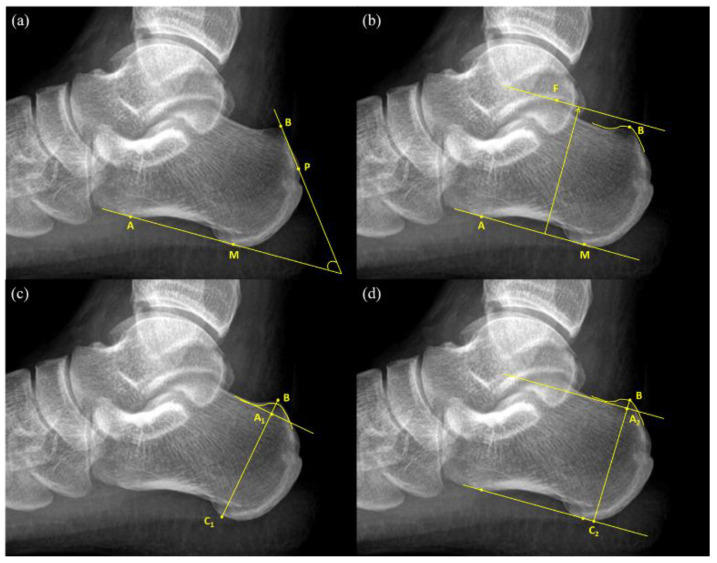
Radiographic measurement of Haglund’s deformity (a) Fowler-Philip angle = Angle between $\bar{\mathrm{AM}\;}$ and $\bar{\mathrm{BP}\;};$ (b) Heneghan-Pavlov parallel pitch lines; (c) Haglund’s height = $\bar{{\mathrm{BA}}_{1}}$; Haglund’s height ratio = $\bar{{\mathrm{BA}}_{1}}/\bar{{\mathrm{BC}}_{1}}$; (d) Bump height = $\bar{{\mathrm{BA}}_{2}}$; bump-calcaneus ratio = $\bar{{\mathrm{BA}}_{2}}/\bar{{\mathrm{BC}}_{2}}$.
